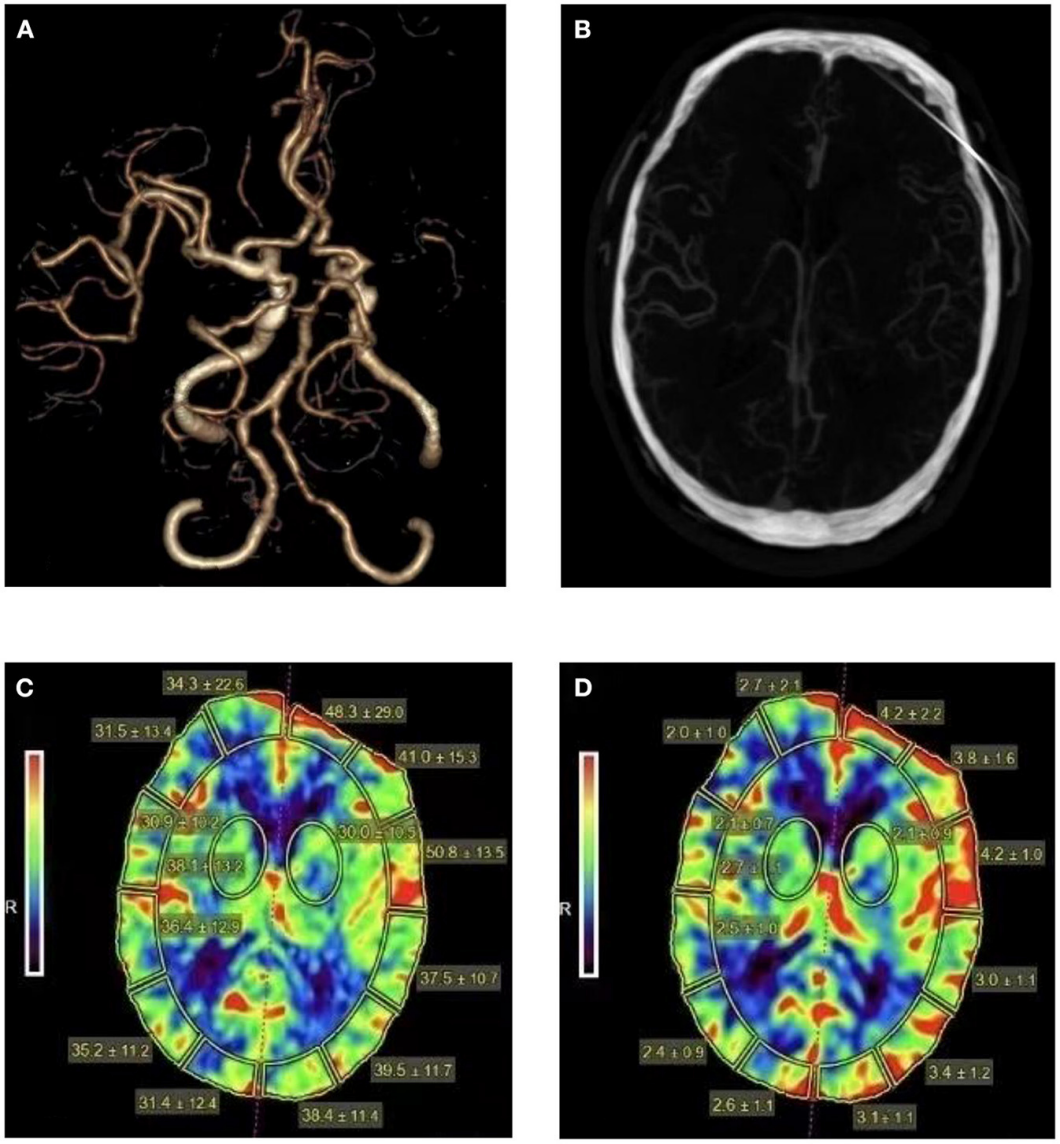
Head CTA, CTA images reconstructed in arterial phase, cerebral blood flow and cerebral blood volume maps for a patient with middle cerebral artery occlusion. A 64-year-old man presented with right limb weakness and was found to have a left MCA stroke with a 90 days mRS of 2 (A). Sufficient collateral vessels can be seen in the occluded MCA territory (B). The CBF (C) and CBV (D) maps show elevated CBF and CBV of the left hemisphere (CBF ratio = 1.18, CBV ratio = 1.45).
